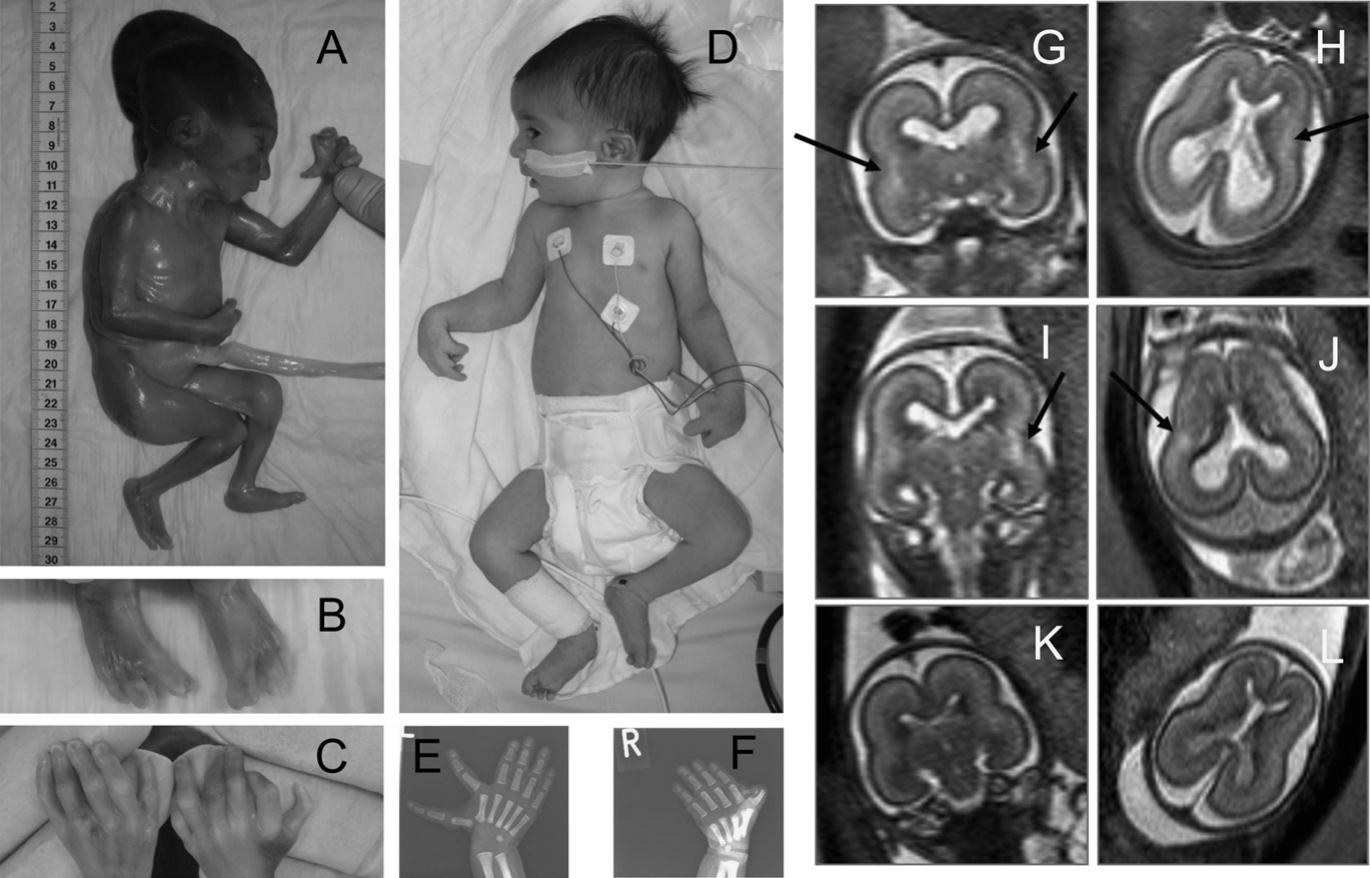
A-F, Clinical manifestation and G-L, CNS abnormalities in fetal magnetic resonance imaging in A-C, G, H, patient 1 and D-F, I, J, patient 2. Note B, E, F, limb malformations consisting of bilateral triphalangeal thumbs, extra preaxial digit on the left hand, and incomplete preaxial polydactyly on the right hand in both patients and C, bilateral cutaneous I/II and III/IV toe syndactyly in patient 1. G, H, T2-weighted fast spin-echo sequences in coronal and axial planes of patient 1, I, J, patient 2, and K, L, a healthy control fetus, each at 21 weeks of pregnancy. Both affected fetuses present with hypoplastic corpus callosum, asymmetric ventricles, and hyperintense T2-weighted signal changes in the region of the triangular crossroads (*arrows* in G-J).
